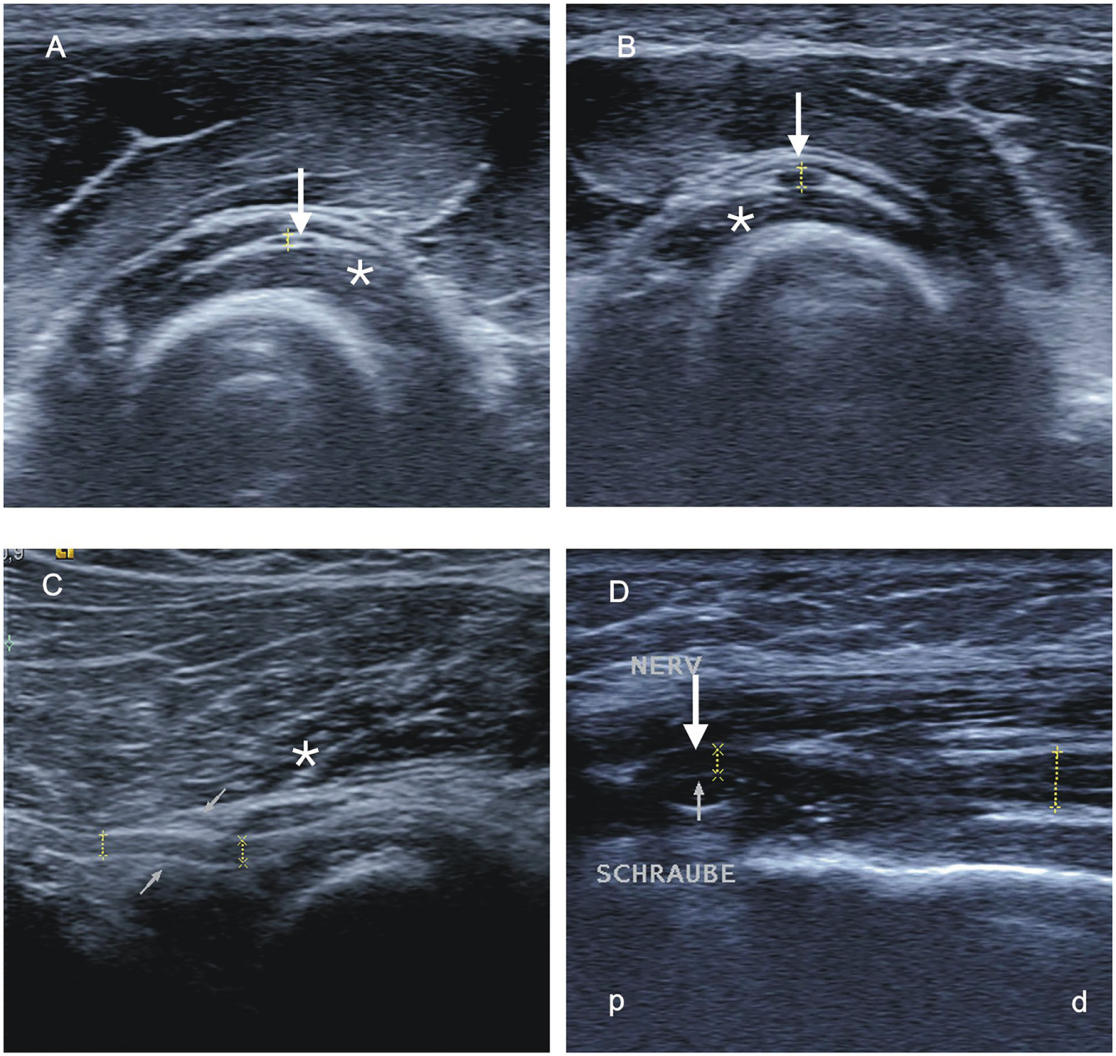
Sonographic studies of a patient with a proximal radial nerve lesion. (A, B) Transversal study of the posterior interosseous nerve (PIN; arrow) within the supinator muscle (asterisk) on the healthy side (A) in comparison to the affected side (B) with a significant swelling of the PIN within the muscle. (C) Longitudinal study of the PIN with a swelling before entering the Arcade of Frohse (thin arrows) and within the supinator muscle (asterisk). (D) Longitudinal study of the radial nerve in the distal upper arm with impression of the nerve (arrow) by a screw (thin arrow). p—proximal, d—distal
